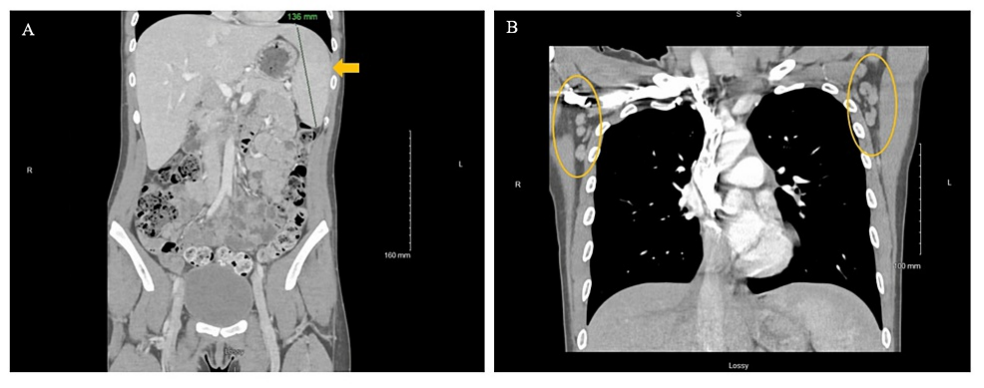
CT of the chest, abdomen, and pelvis Note: Shown are the coronal images of the abdomen (Panel A) obtained with soft tissue windows from CT performed with the administration of intravenous contrast material, showing mild splenomegaly, the spleen measuring 13.6 cm, without hepatomegaly. Coronal soft tissue CT imaging (Panel B) of axillary lymph nodes was unremarkable. No prior imaging was available for comparison. These findings are less consistent with suggested malignancy through the chest, abdomen, or pelvis. No other acute abnormalities were seen.
